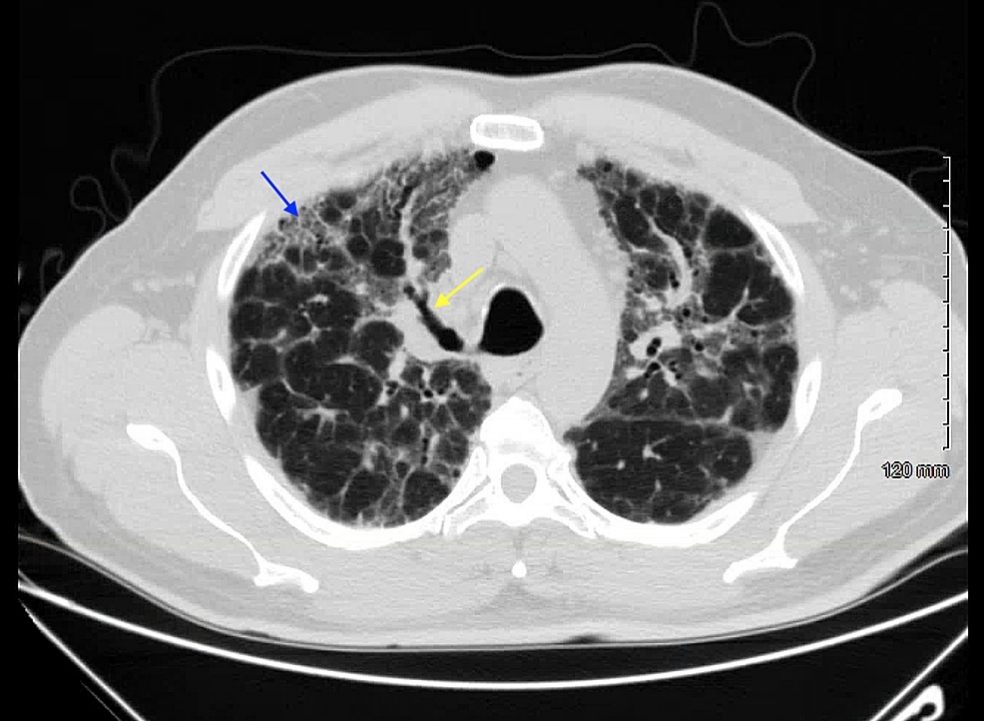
High-resolution computed tomography depicting ground-glass opacities (blue arrow) and bronchiectasis (yellow arrow) at the level of the aortic arch
